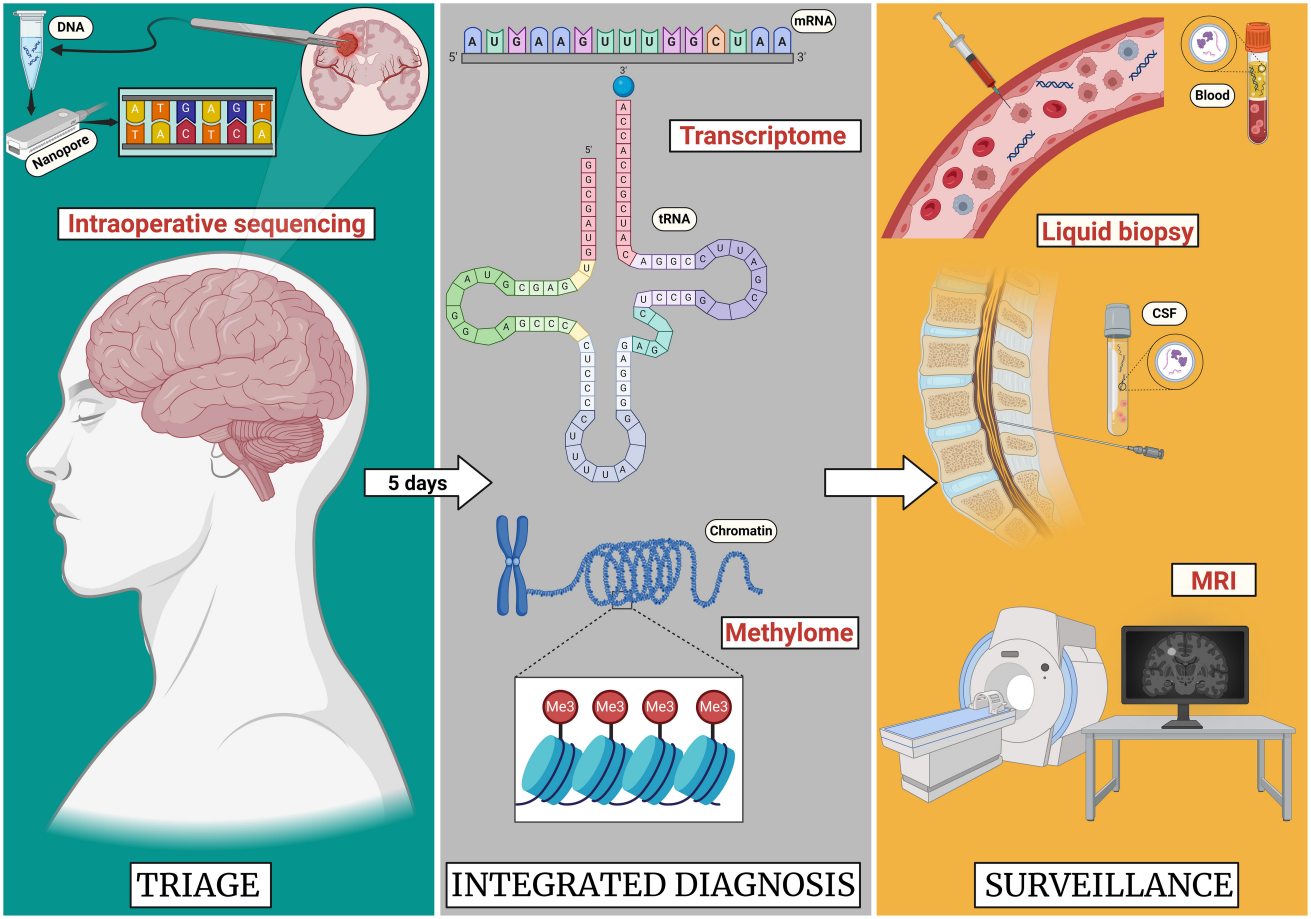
Proposed future paradigm of long read sequencing in the application of CNS tumors. As the sequencing technologies improve, the advances in the neurooncological practice will evolve. In the case of a brain tumor resection, these sequencing technologies could allow for the intraoperative diagnosis of the tumor allowing for decision making on extent of resection during the surgery. While in 5 days a complete transcriptome and methylome profile of the tumor could be obtained providing improved prognostication. Lastly follow-up surveillance will likely include LRS for liquid biopsy in combination with standard MRI surveillance. Created with Biorender.com.
